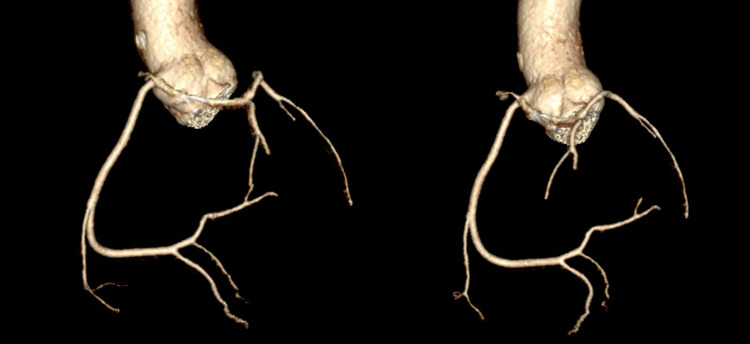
Coronary computed tomography angiogram with 3D reconstruction of the single coronary artery Coronary computed tomography angiogram with 3D reconstruction showing the single coronary artery arising from the right coronary cusp then separated into the right coronary artery and left coronary artery.
